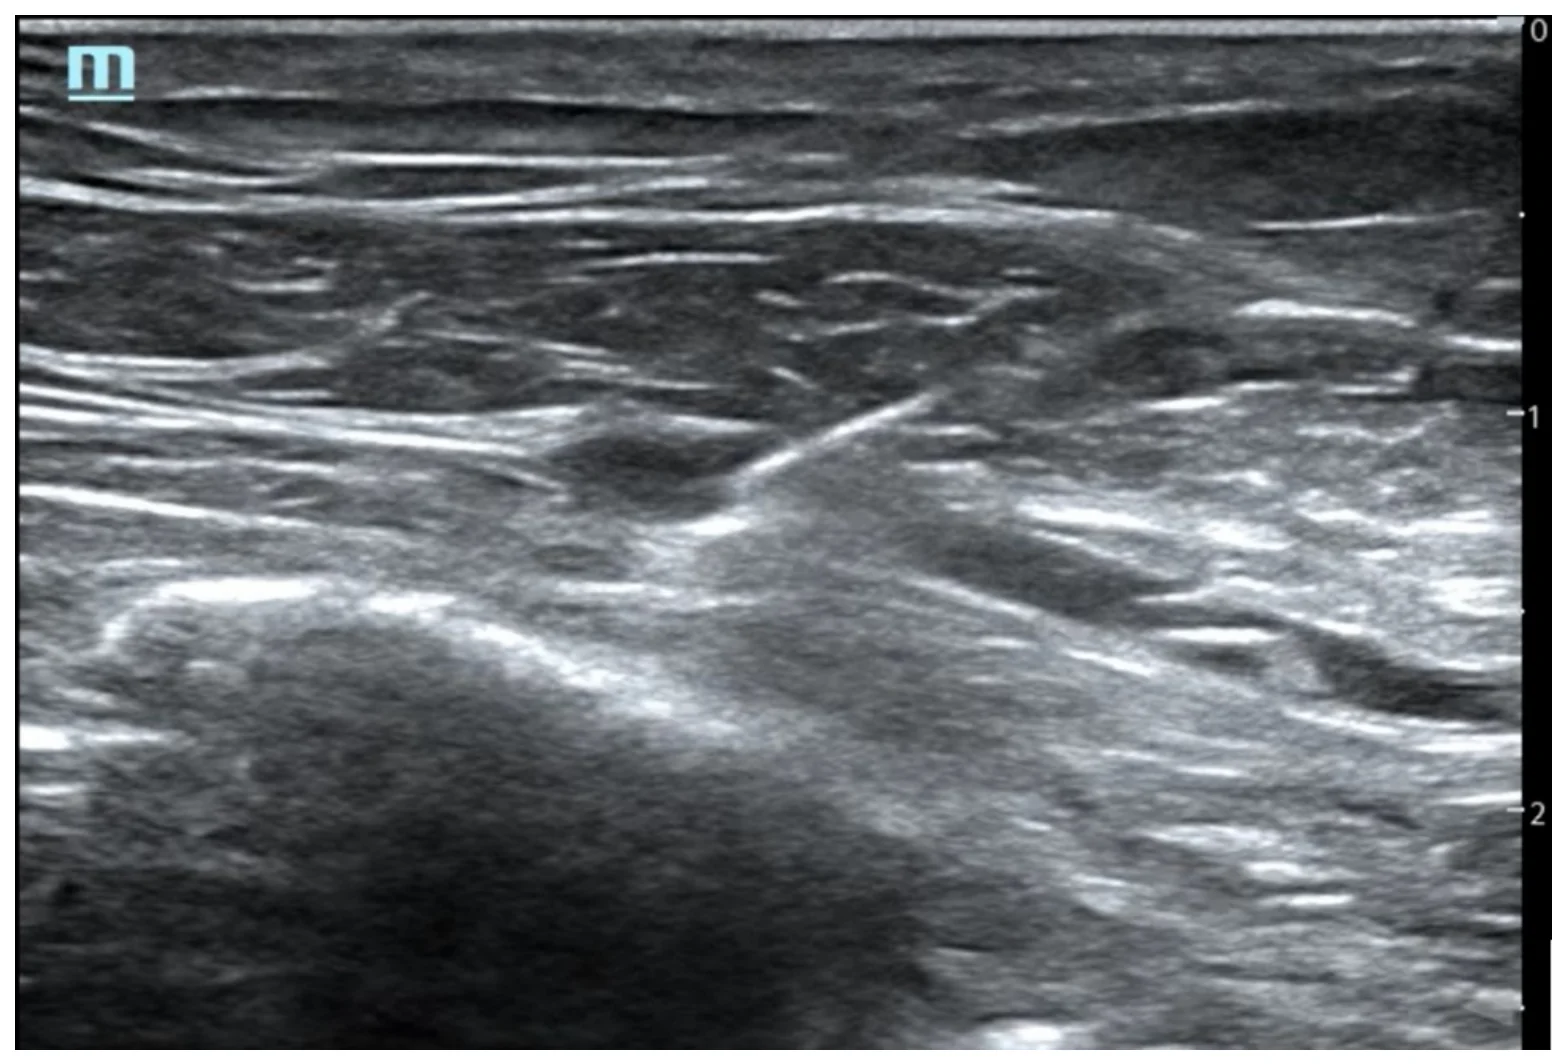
Ultrasound view of hydrodissection during PECS I block.
